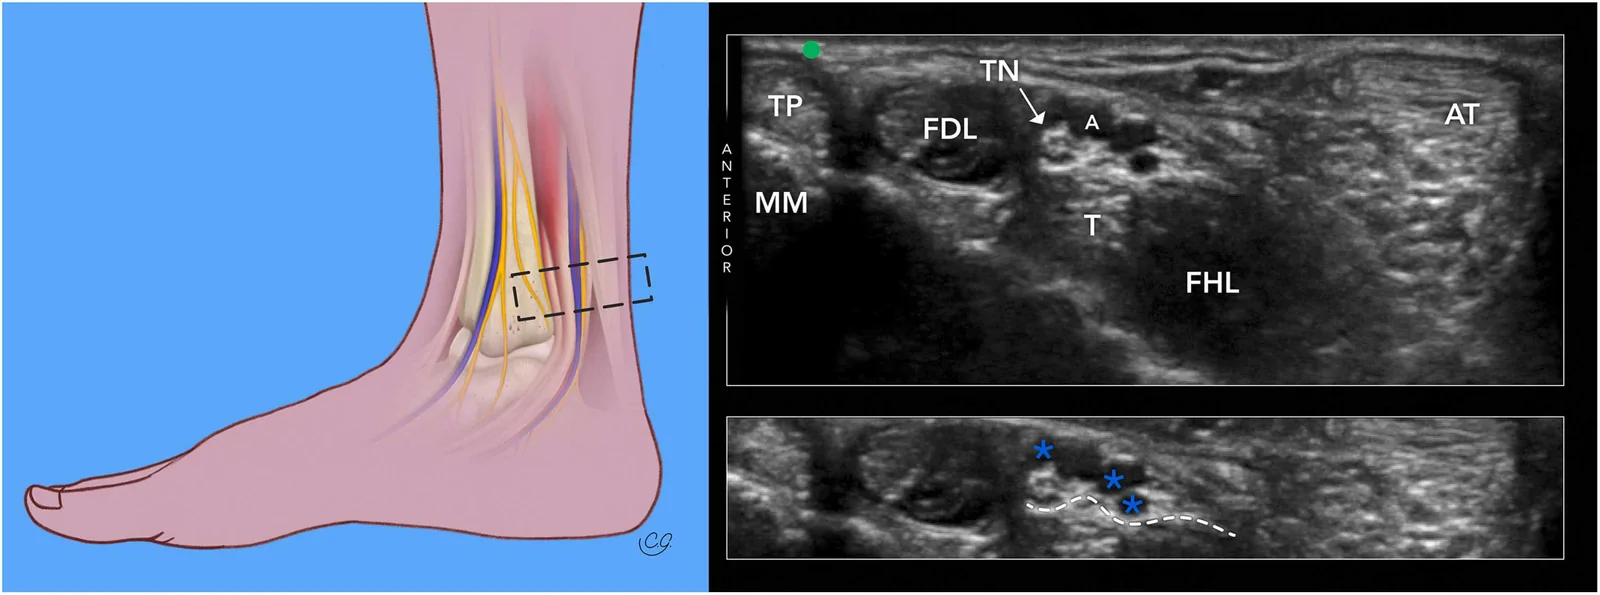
Ultrasound image and corresponding probe position for the tibial nerve block. A: tibial artery, AT: Achilles tendon, FDL: flexor digitorum longus muscle and tendon, TP: tibialis posterior tendon, FHL: flexor hallucis longus muscle and tendon (T), MM: medial malleolus, TN: tibial nerve, dotted white line: flexor hallucis longus fascia, *: veins accompanying the tibial artery. Please note that the flexor retinaculum over the tibial nerve and neurovascular bundle was removed to show the underlying tibial nerve. Reproduced with permission from Girón Arango Medicine Professional Corporation.
